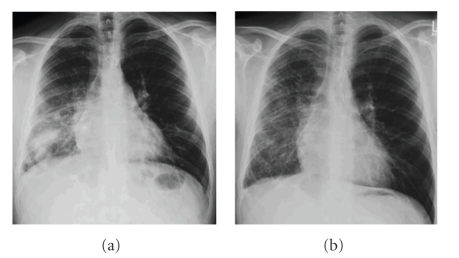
Chest radiographs (a) at presentation in GUCH clinic and (b) after several weeks of antibiotic treatment. Right pulmonary venous obstruction is suggested by the presence of dilated venules, Kerley lines and fluid in the fissures, all more obvious after the consolidation was treated.
